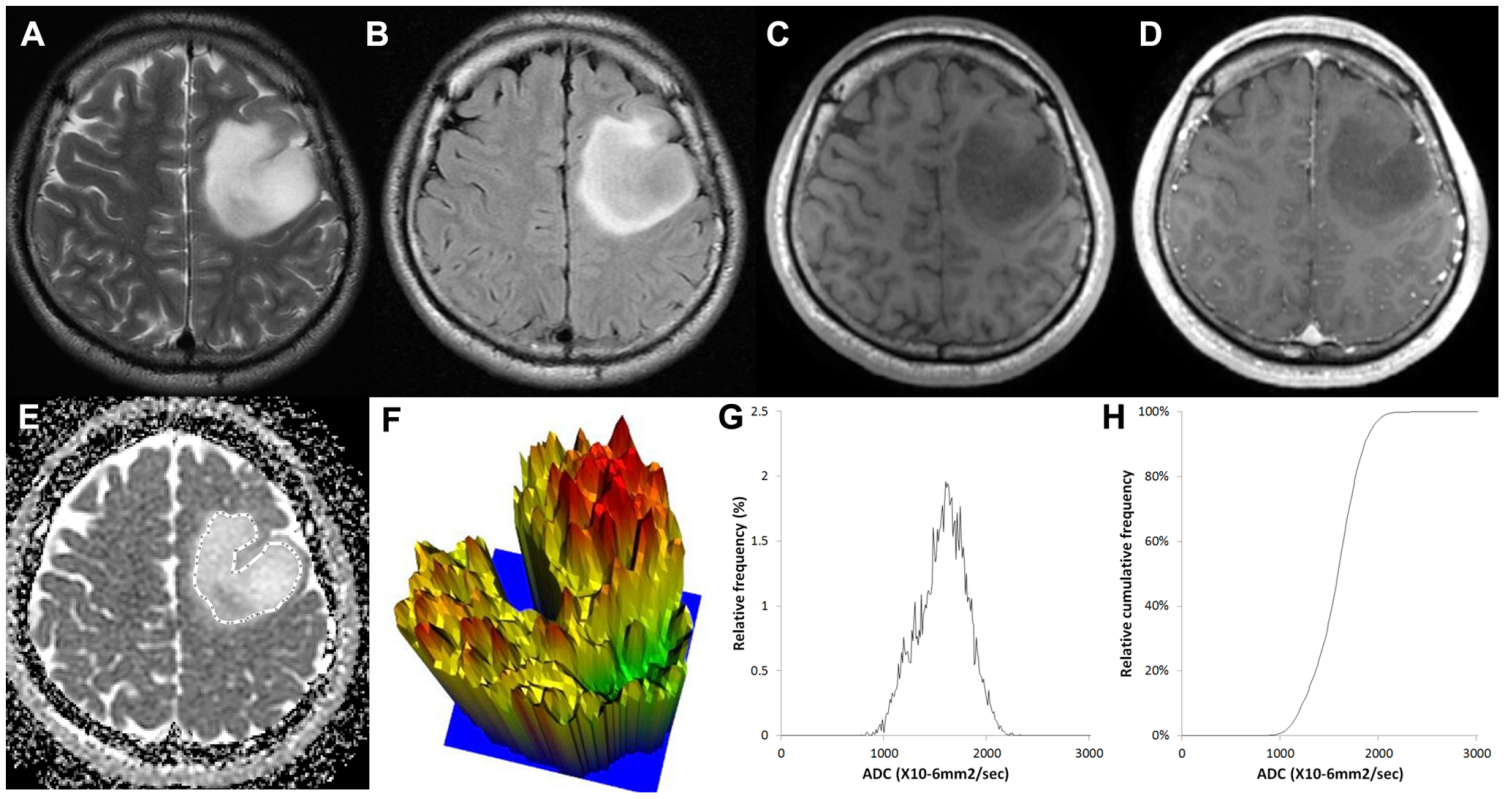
Images of a 30-year-old male with a grade III anaplastic astrocytoma. (A) T2-weighted image, (B) T2 FLAIR image, (C) T1-weighted image, (D) contrast-enhanced T1-weighted image, (E) ADC map with ROI placement, with the corresponding (E) 3-D height map of the ADC signal intensity, (G) histogram of ADC and (F) cumulative ADC histogram. The entropy value of ADC was 6.792.
